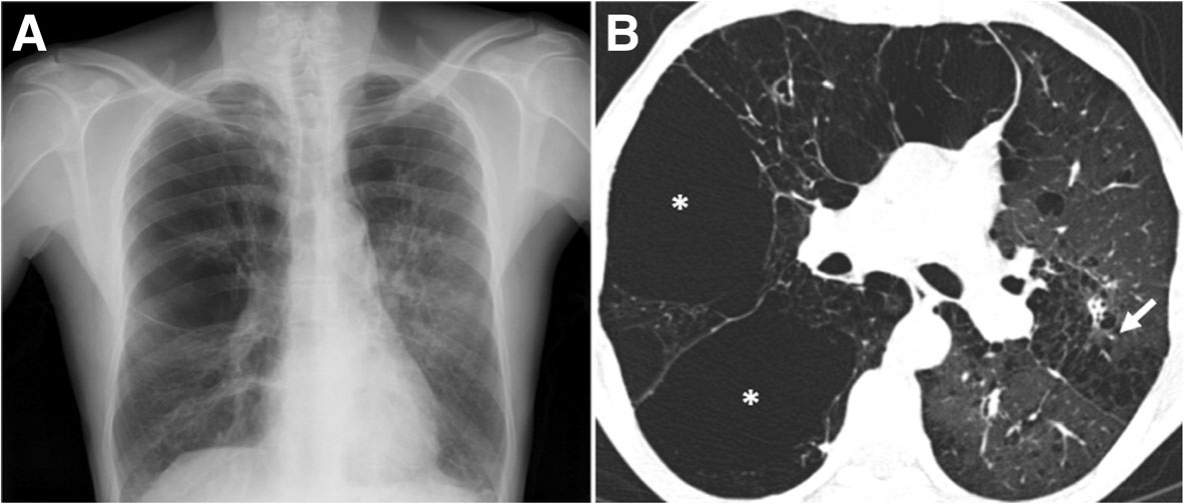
Bullous emphysema in a 55-year-old man. a Posteroanterior (PA) radiograph shows lucencies in the superior half of the right hemithorax and in the upper left hemithorax. b Axial CT shows upper lobe predominant bullae (asterisks) in the subpleural surface of the right lung. Moderate CLE is also present (arrow)
